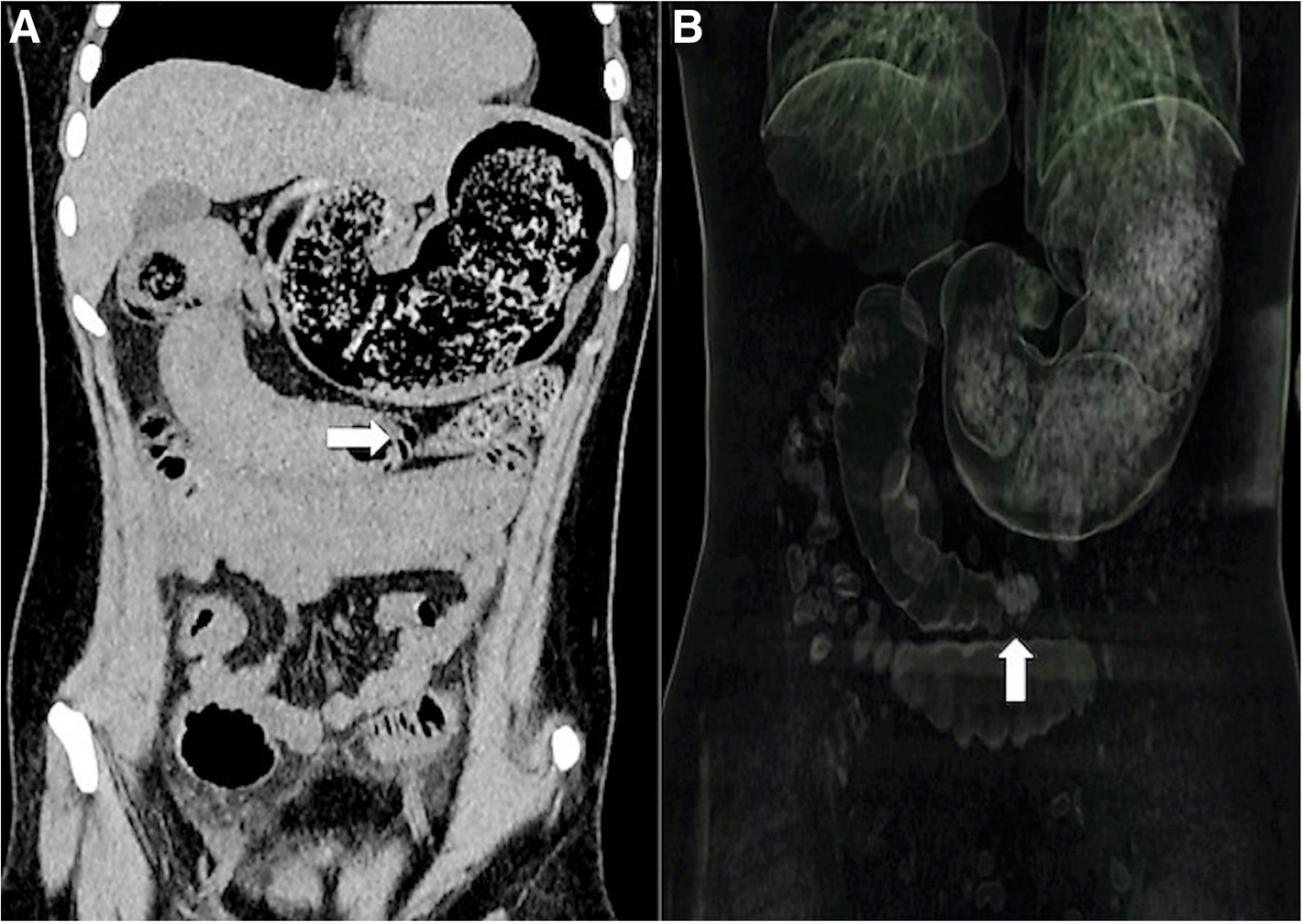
a Computed tomography with coronal reconstruction, we observe a beehive pattern within the gastric chamber that extends into duodenum, observing a transition zone (*arrow*) in the proximal jejunum. b Volumetric reconstruction, observing an image suggesting intussusception (*arrow*)
